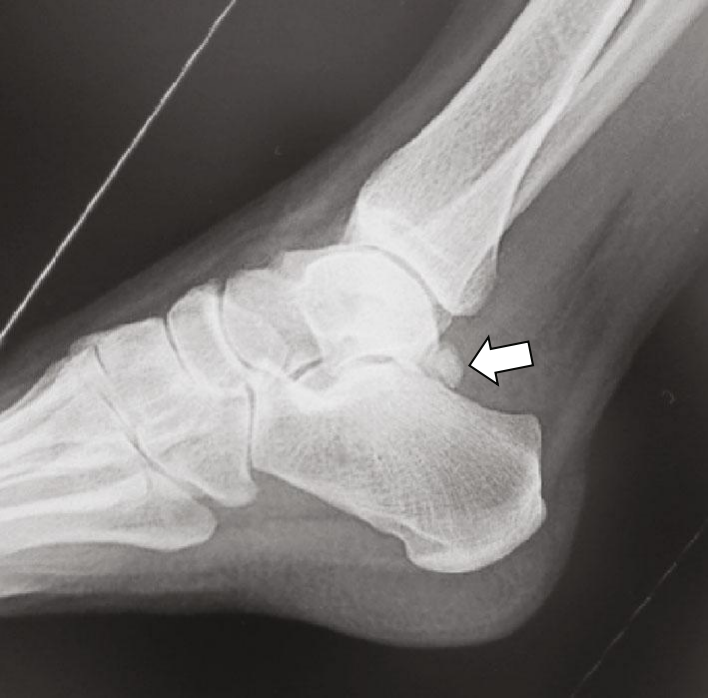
Lateral radiograph showing the os trigonum (arrow).
